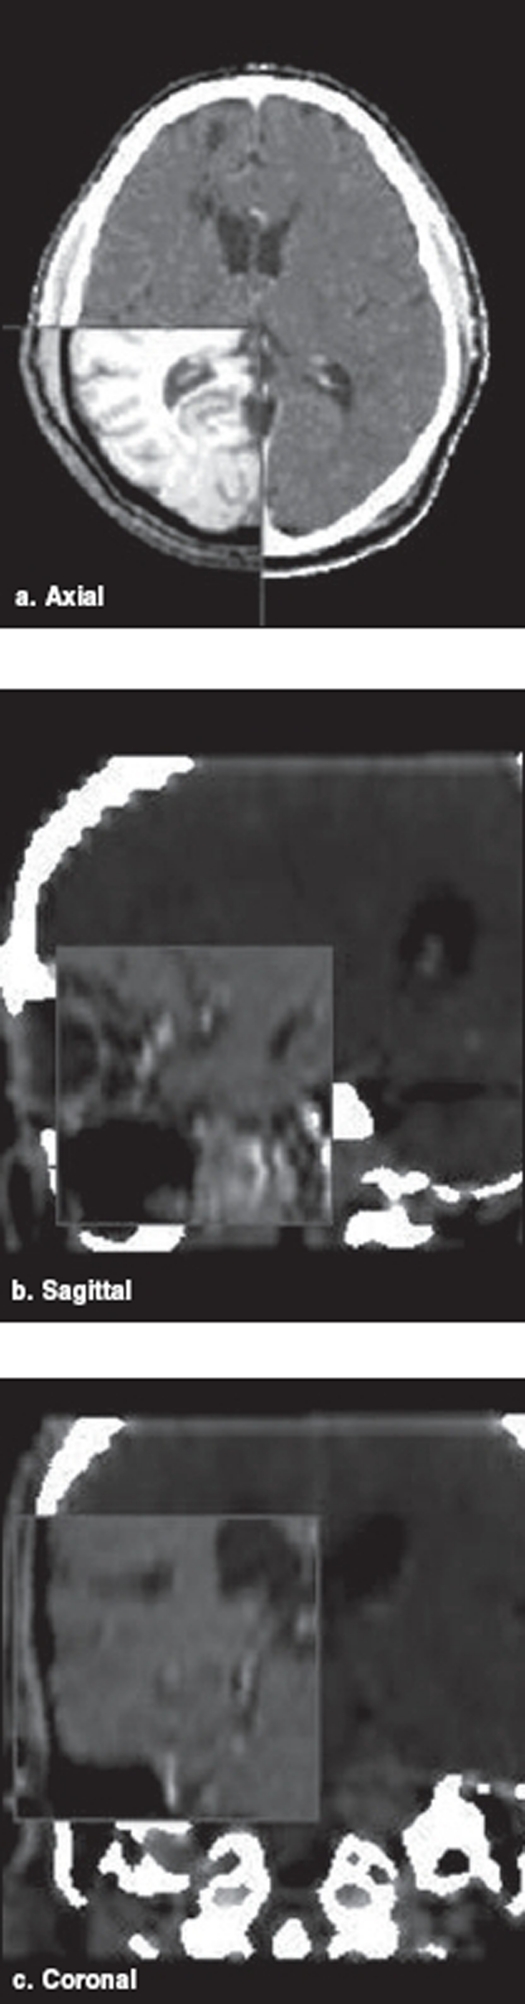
Verification of X-ray CT and MRI image sets along various planes
